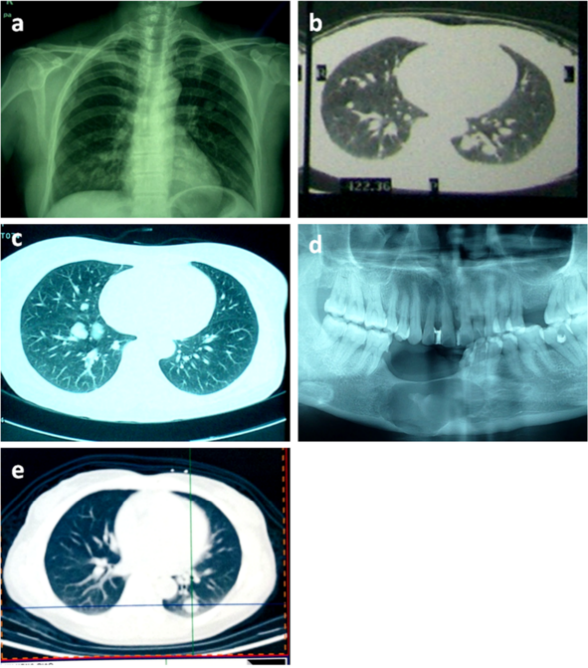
X-ray and CT findings of MA. (a) and (b): X-ray and CT showing bilateral pulmonary nodules in patient 1. (c): CT scan showing bilateral pulmonary nodules that are mainly located in the right lung in patient 2. (d): X-ray of recurrent ameloblastoma in patient 2 showing a cystic radiolucency with scalloped and hyperostotic borders below the former surgical region involving the inferior border of mandible. Expansile destruction of the inferior mandibular cortex was seen. (e): CT scan showing bilateral pulmonary nodules in patient 3. The lobular or irregular round nodules with invariable sizes in all the three cases
